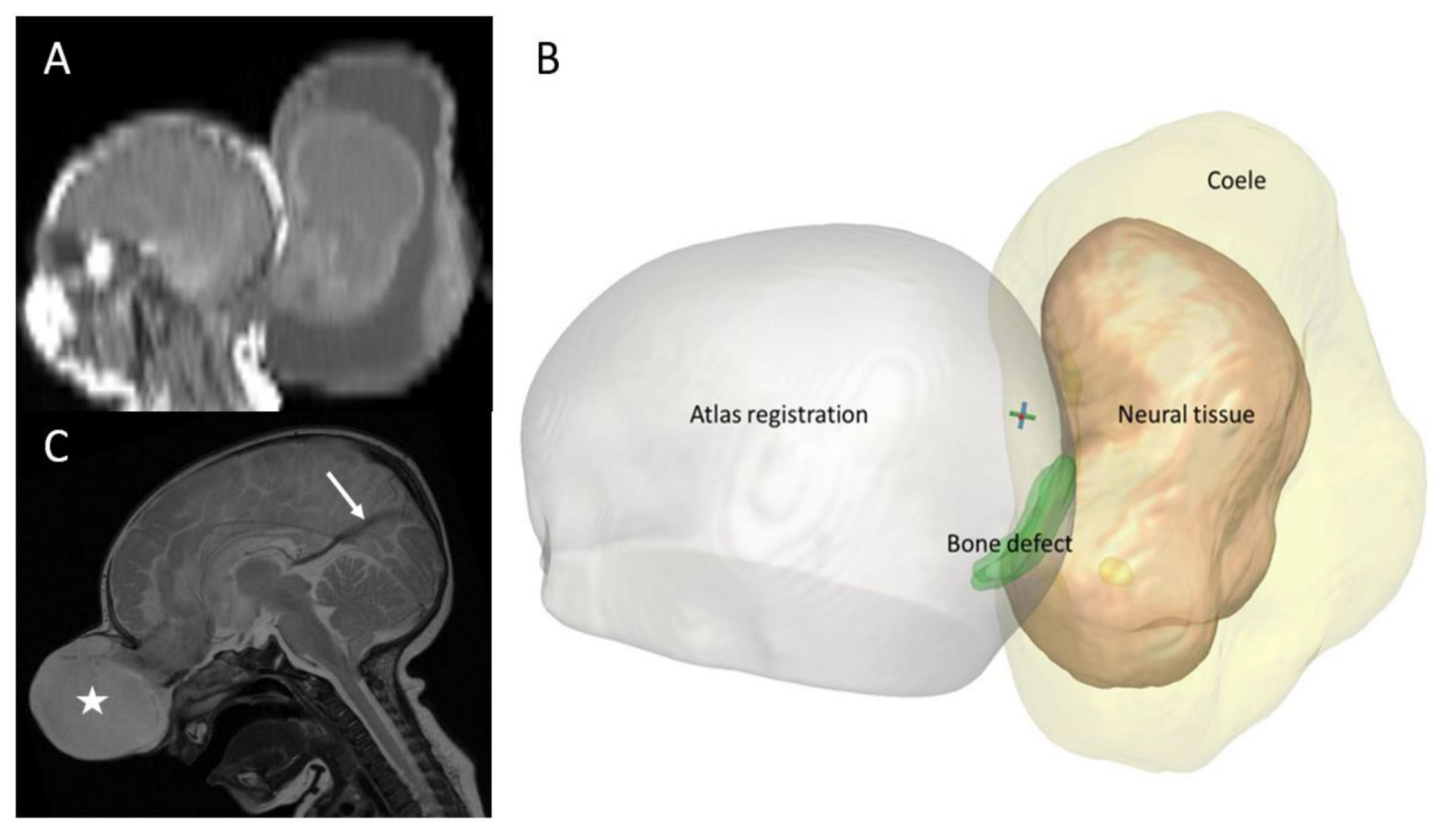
Example dataset findings. Example patient, A) post-natal sagittal MRI depicting a large peri-torcular encephalocele, B) 3D volumetric segmentation showing the bone defect (green), neural tissue (beige) and total encephalocele (yellow) after non-linear transformation to MNI-atlas space. C) Sagittal MRI image from a different patient with a falcine venous sinus (arrow) associated with an anterior skull base encephalocele (star).
